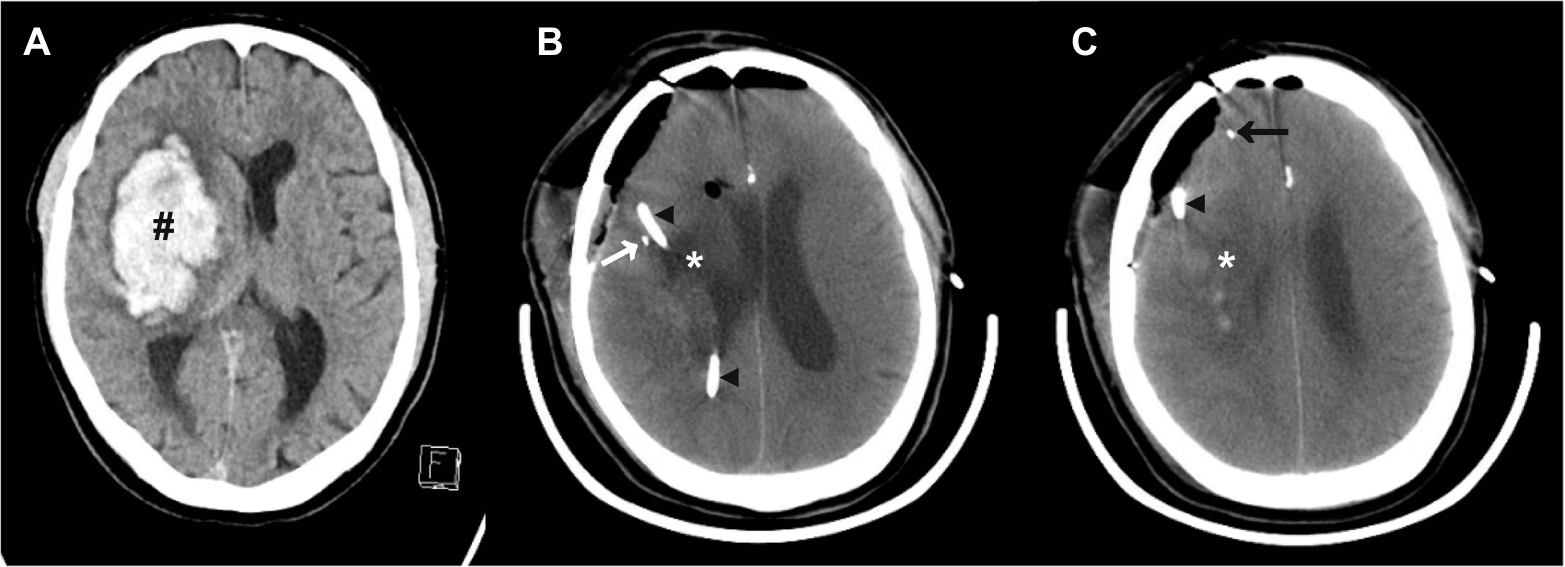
a Preoperative Computed Tomography (CT) scan of patient 1 showing the intracerebral hemorrhage (#). b-c Post-operative CT scan showing the hematoma cavity (*), the perihemorrhagic microdialysis catheter (PHZ; white arrow), c the catheter in seemingly normal cortex (SNX; black arrow) and the external ventricular drain (black arrow head;◄)
